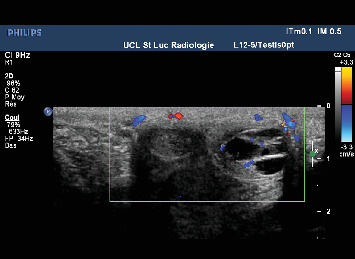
Doppler ultrasound of the penis: heterogeneous and oval formation measuring 15 × 9 mm with peripheral and septal vascularisation inside the left corpus cavernosum.
